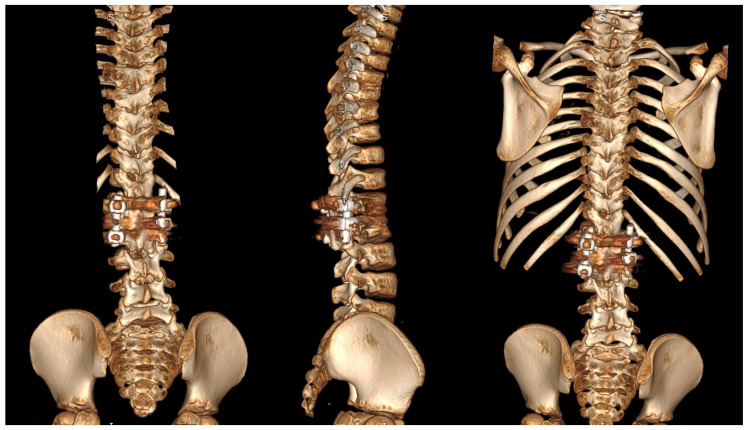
3D CT of 3-year-old boy with congenital scoliosis caused by additional hemivertebrae in thoracic and lumbar spine, who underwent hemivertebrectomy in lumbar spine from posteriori approach only with short posteriori spinal fusion. 3D CT shows spine at 4-year follow-up. It should be noted that there is also a hemivertebra in the thoracic region; however, it is stable and has not shown any progression of the curvature, so surgical treatment has not been required to date.
